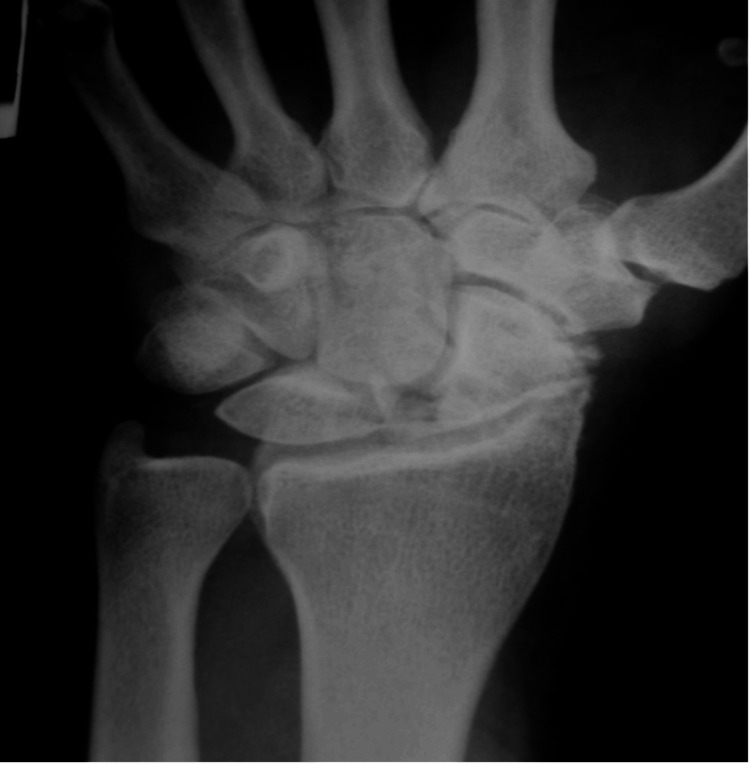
Wrist radiograph showing sclerosis and narrowing of the radioscaphoid articulation, with widening of the scapholunate (SL) interval - Stage II SLAC. Source: Dr. Emmanouil Apergis. SLAC: Scapholunate advanced collapse.
